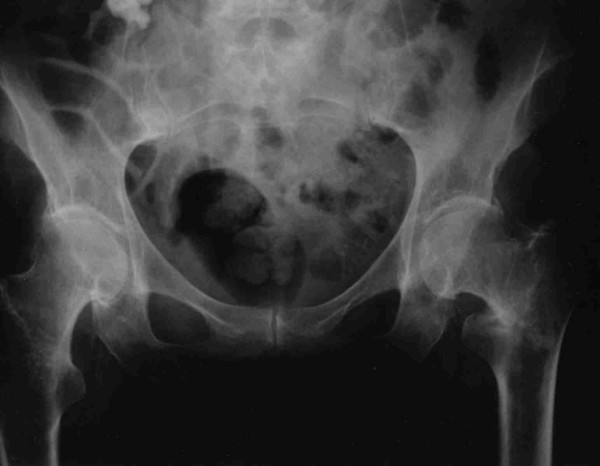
AP radiograph of pelvis.
